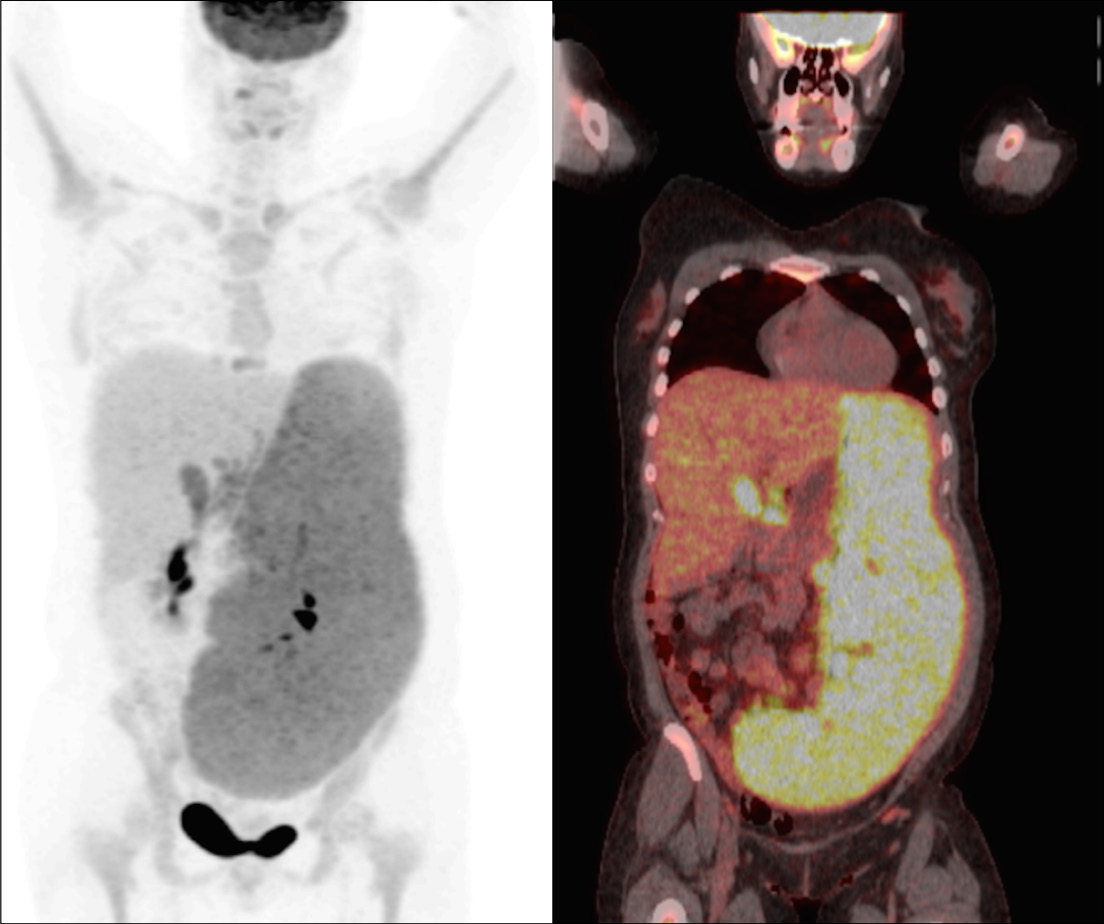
18FDG-PET-CT (fludeoxyglucose F18 position emission computed tomography) demonstrating major splenomegaly (standard uptake value [SUV]: 6.1) and hepatomegaly (SUV: 3.2)
